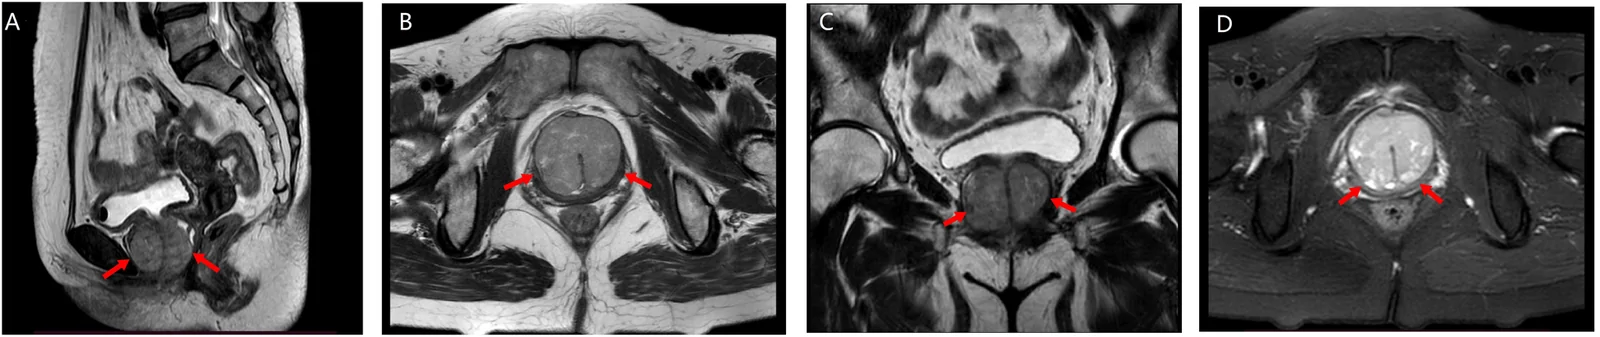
MRI showing a mass, with abnormal signal shadow around the urethra, approximately 4.8 cm × 4.4 cm × 3.9 cm in size, with the tendency to grow around the urethra (A–C). The DWI sequence shows high signal, and the enhanced scan shows mild uneven enhancement, with a shape similar to that of the prostate (D).
